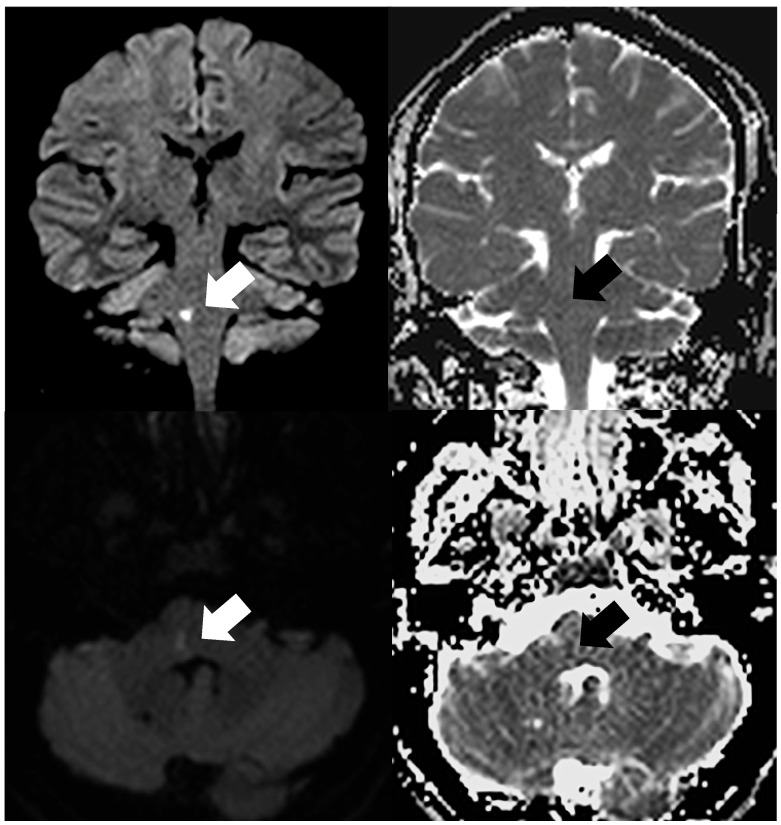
Acute ischemic lesion in the pons, specifically the right middle cerebellar peduncle. Upper row: coronal DWI (b = 1000 s/mm^2^) and corresponding ADC map; lower row: axial DWI (b = 1000 s/mm^2^) and corresponding ADC map. The lesion is indicated by a white arrow on DWI and a black arrow on ADC. Lesion conspicuity is greater on coronal images compared with axial images.
